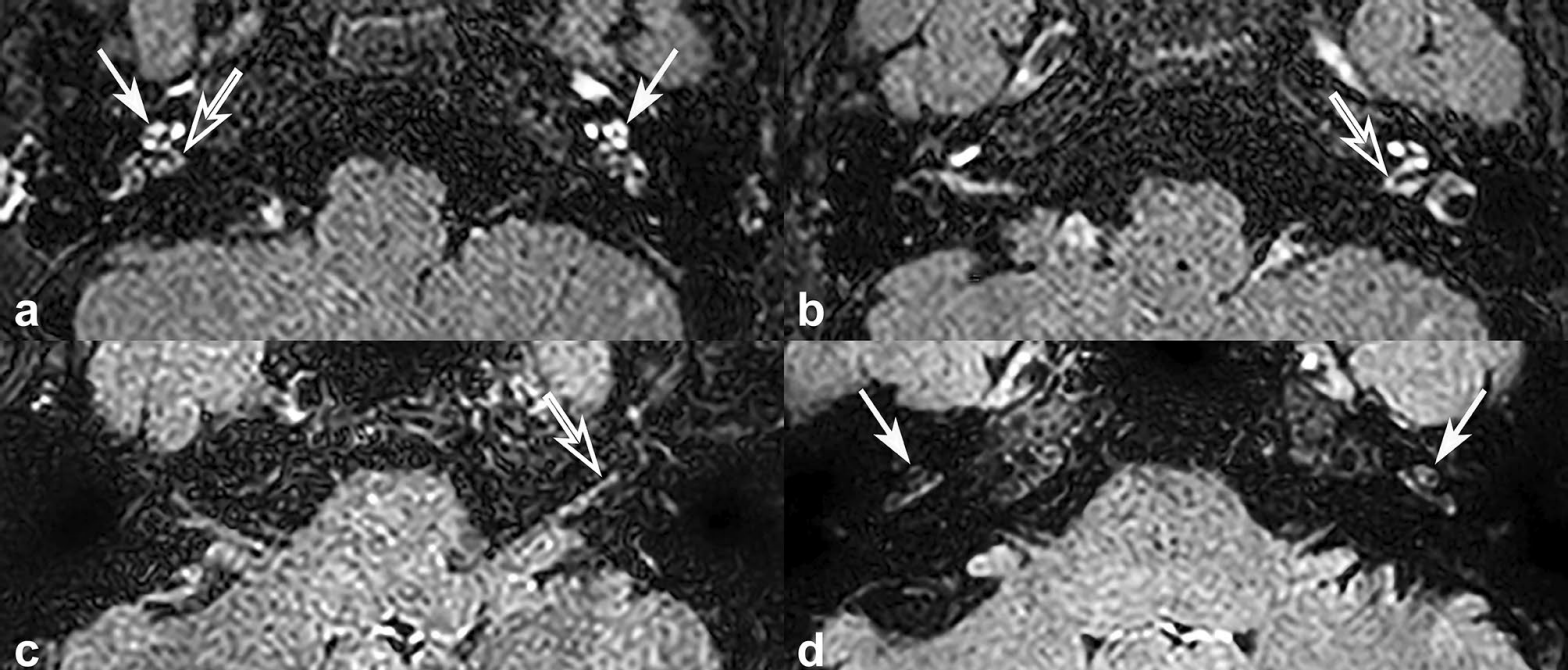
Representative cases of CINCA syndrome. (a,b) A 13-year-old male diagnosed with CINCA syndrome presented with intense inner ear enhancement in bilateral cochlea (arrows) and strong enhancement of bilateral cochlear nerves (empty arrows) on post-contrast FLAIR image (postFLAIR). (c) A 42-year-old female diagnosed with CINCA syndrome presented with strong enhancement of left cochlear nerve on postFLAIR (empty arrow) compared to enhancement of the cochlea. (d) A 38-year-old female diagnosed with CINCA syndrome presented with postFLAIR-enhancement of bilateral cochlea (arrows), but absence of enhancement of the cochlear nerve.
